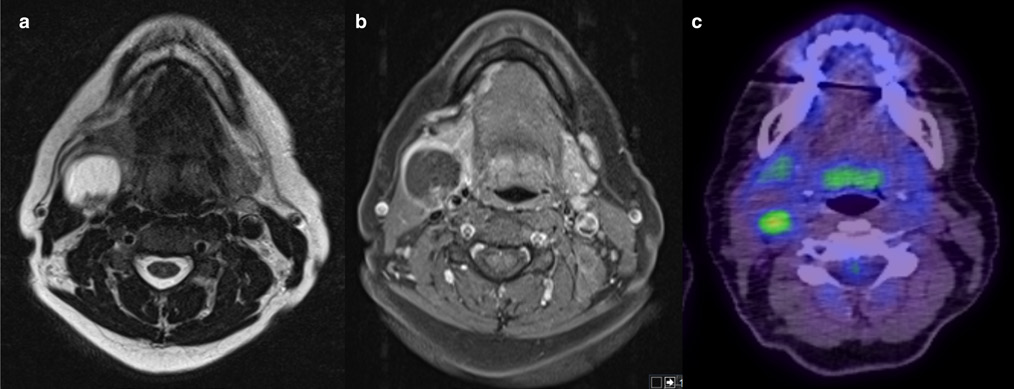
Cystic neck mass evaluation with MRI &^18^F FDG PET-CT. Differentiation between branchial cleft cysts and cystic metastases in HPV+ OPC with an occult primary can be challenging. However, features such as a solid, enhancing mural nodule (seen on axial *T*
